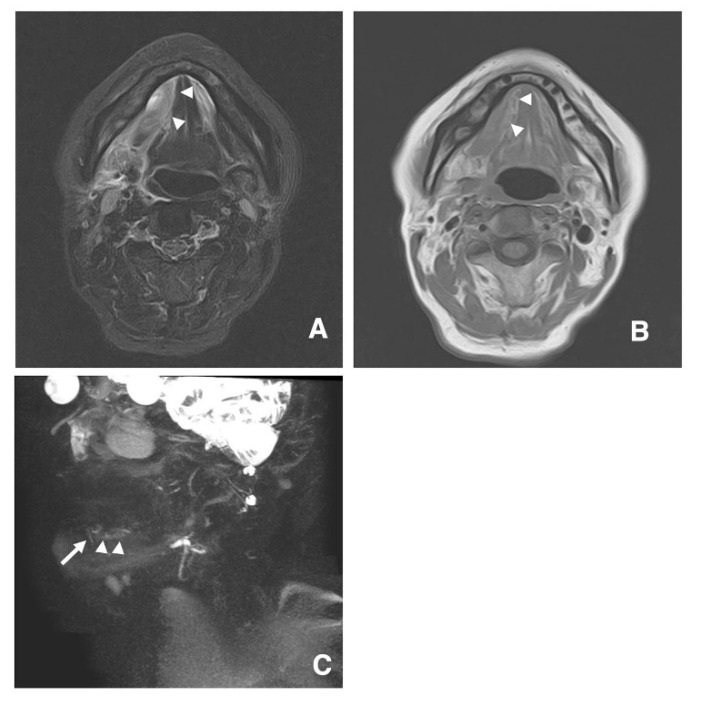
STIR (A), T1-weighted images (B), and MR sialography (C) of a 76-year-old man with inflammation of the right oral floor. The disappearance (arrows) of many sublingual gland ducts in continuity with the sublingual glands is visualized using MR sialography.
